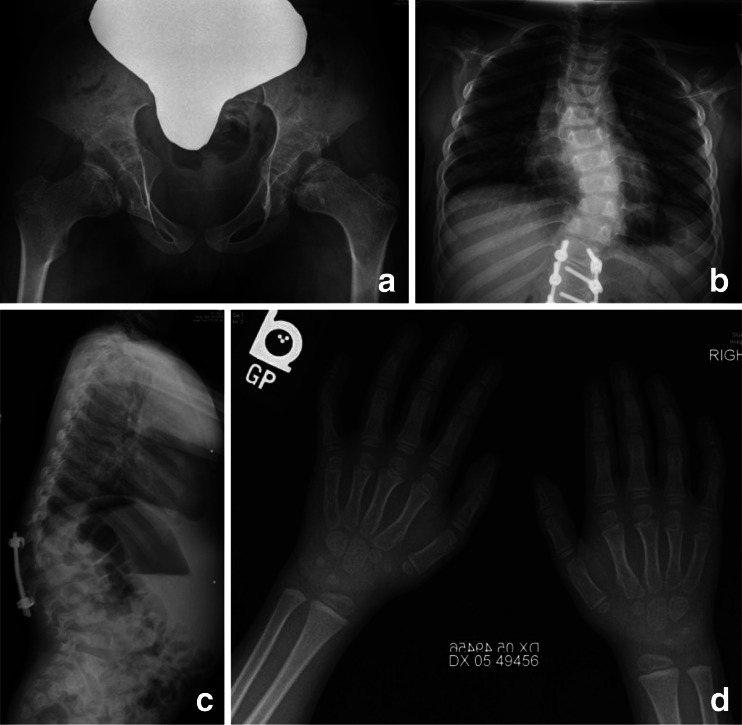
Radiographs of patient 4, female, MPS IVA. a AP pelvis and hips, age 9 years: dysplastic CFE; mildly tapered lower iliac wings merging into the acetabular roofs. b AP spine and ribs, age 9 years: severe scoliosis with hardware; normal ribs. c Lateral spine, age 2½ years: gibbus; hypoplastic T12; middle beaked L1. d AP hands and wrists, age 6 years: relatively normal, but carpal ossification delay
